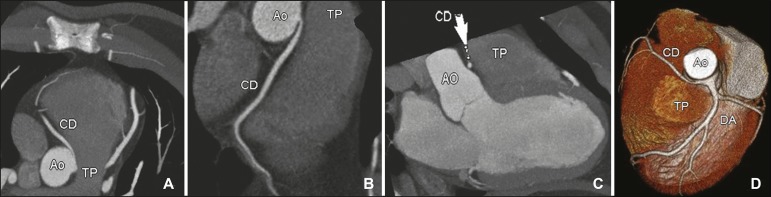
Contrast-enhanced axial CT (A), oblique reconstructions
